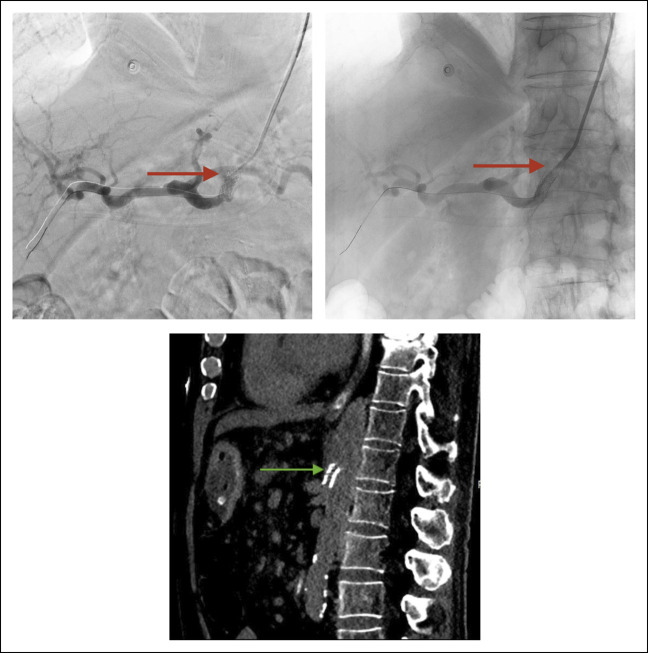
Control angiography after stent placement in the celiac trunk stenosis (red arrows) with (left side image) and without (right side image) bone subtraction. Intra-procedural CT was performed with sagittal reformat (bottom image) to confirm correct stent placement covering the celiac trunk stenosis into the aorta.
